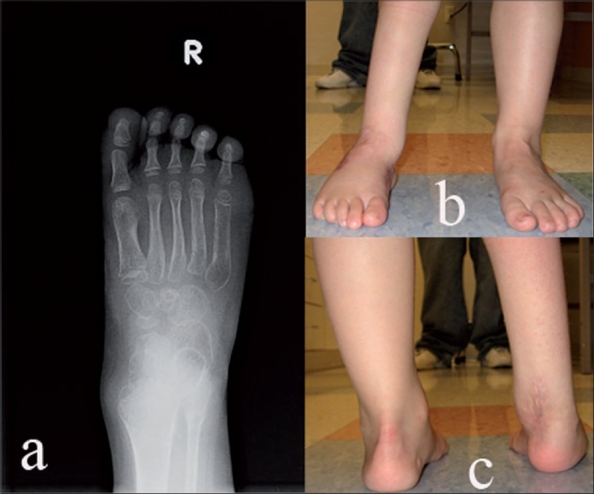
Clinicoradiological deformity correction of the same patient after treatment with manipulation, Achilles tendon lengthening and tibialis anterior transfer. (a) Postoperative AP X-ray of foot with ankle depicting correction of forefoot adduction. (b,c) Clinical appearance of right foot after treatment
